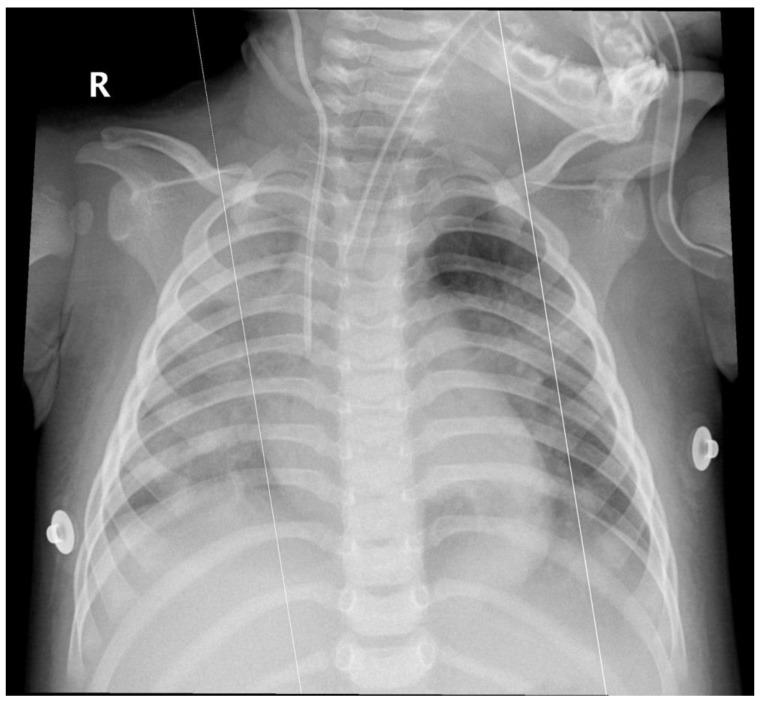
Chest X-ray obtained after intubation, showing diffuse bilateral pulmonary infiltrates.
